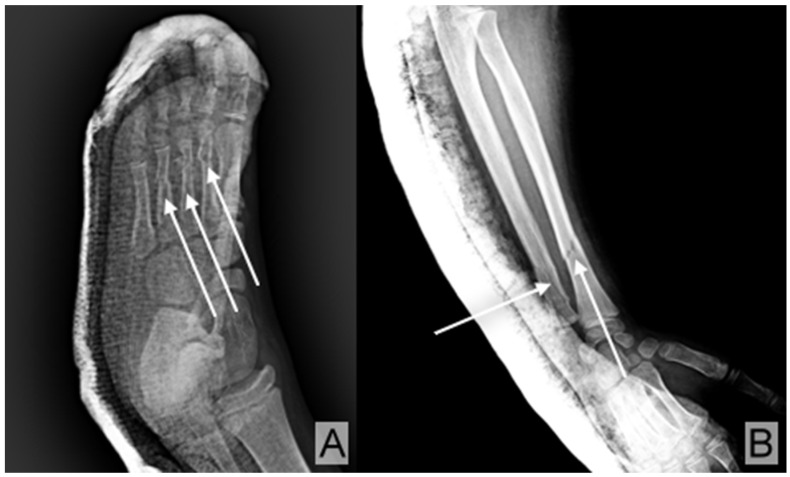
(A) X-ray of the right leg showing open fractures (arrows) of the second, third, and fourth metatarsals. (B) X-ray of the right forearm revealing fractures (arrows) of both the radius and ulna.
